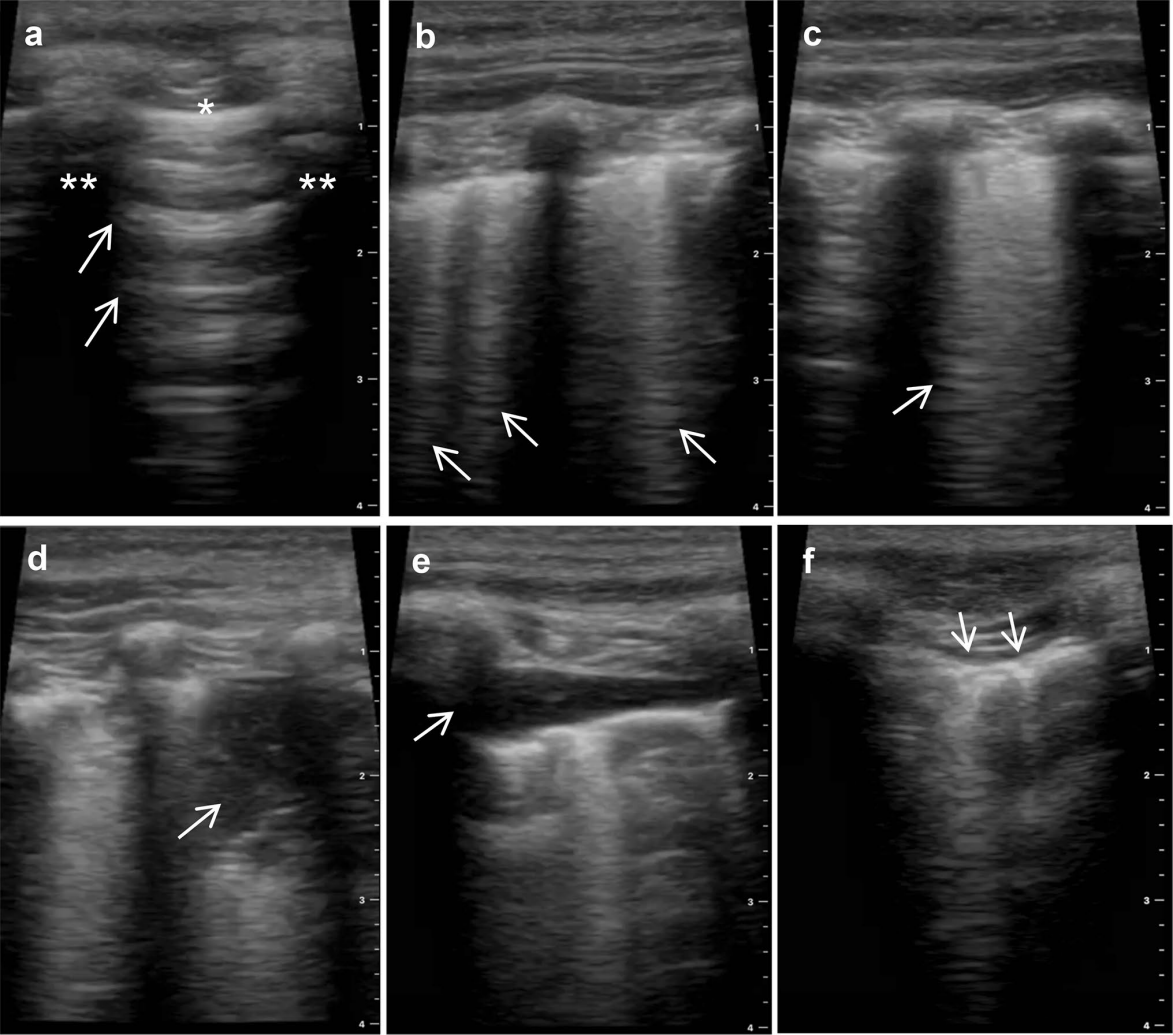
Lung ultrasound patterns. a A lines (*arrows*), reverberation artefacts of the pleural line (*) between two rib shadows (); b Isolated B lines (*arrows*), pathological signs of interstitial pulmonary oedema; c Multiple coalescing B lines (*arrow*); d Consolidation (*arrow*) showing characteristic tissue-like pattern (i.e. “lung hepatisation”) and air bronchograms; e Pleural effusion (*arrow*); f Sub-pleural nodules (*arrows*)
